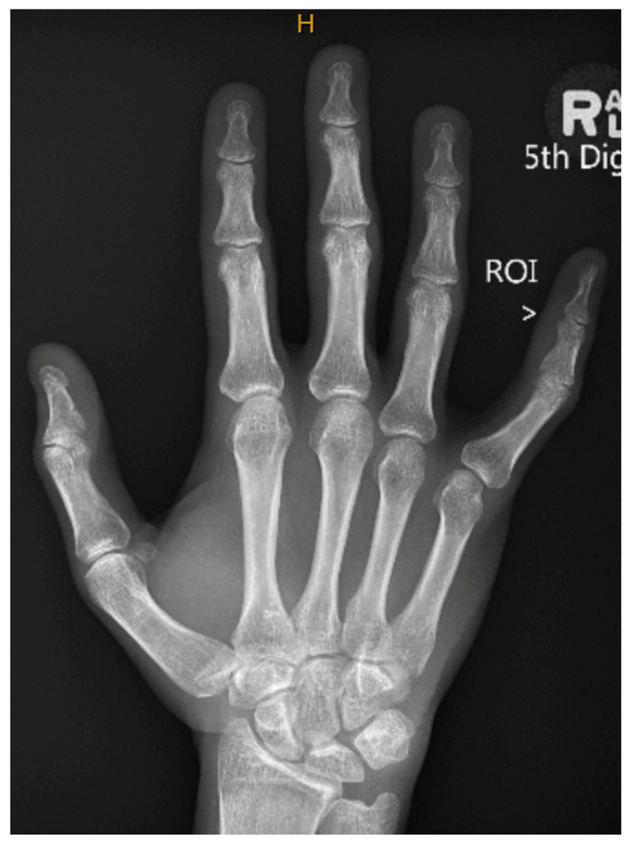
Plain Radiography of the Right Little Finger.
